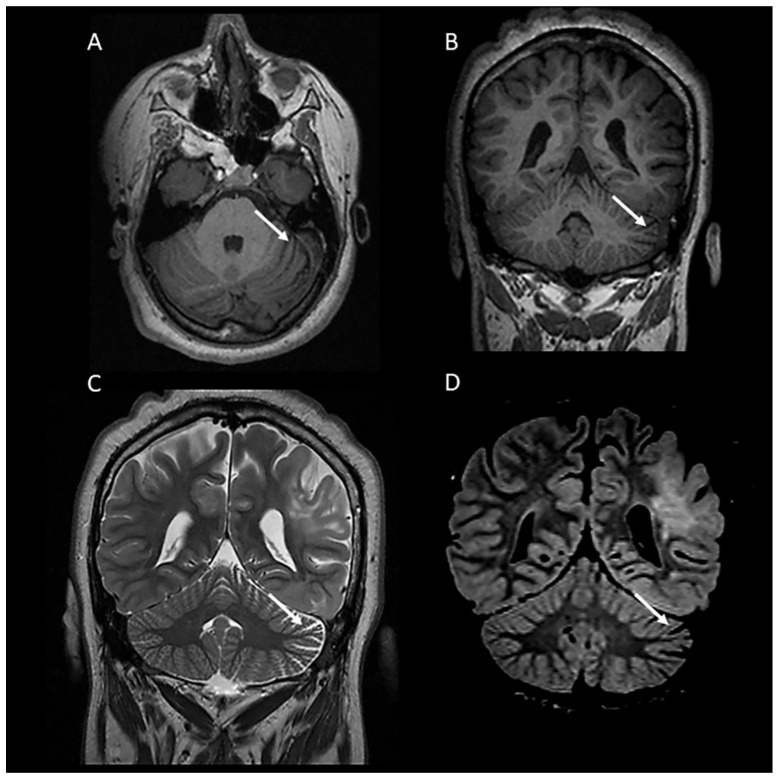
A 19-year-old female with tuberous sclerosis complex. Axial (A) and coronal (B) T1-weighted and coronal T2-weighted (C) and FLAIR (D) MR images demonstrating left hemi-cerebellar atrophy characterized by unilateral widening of the cerebellar fissures.
